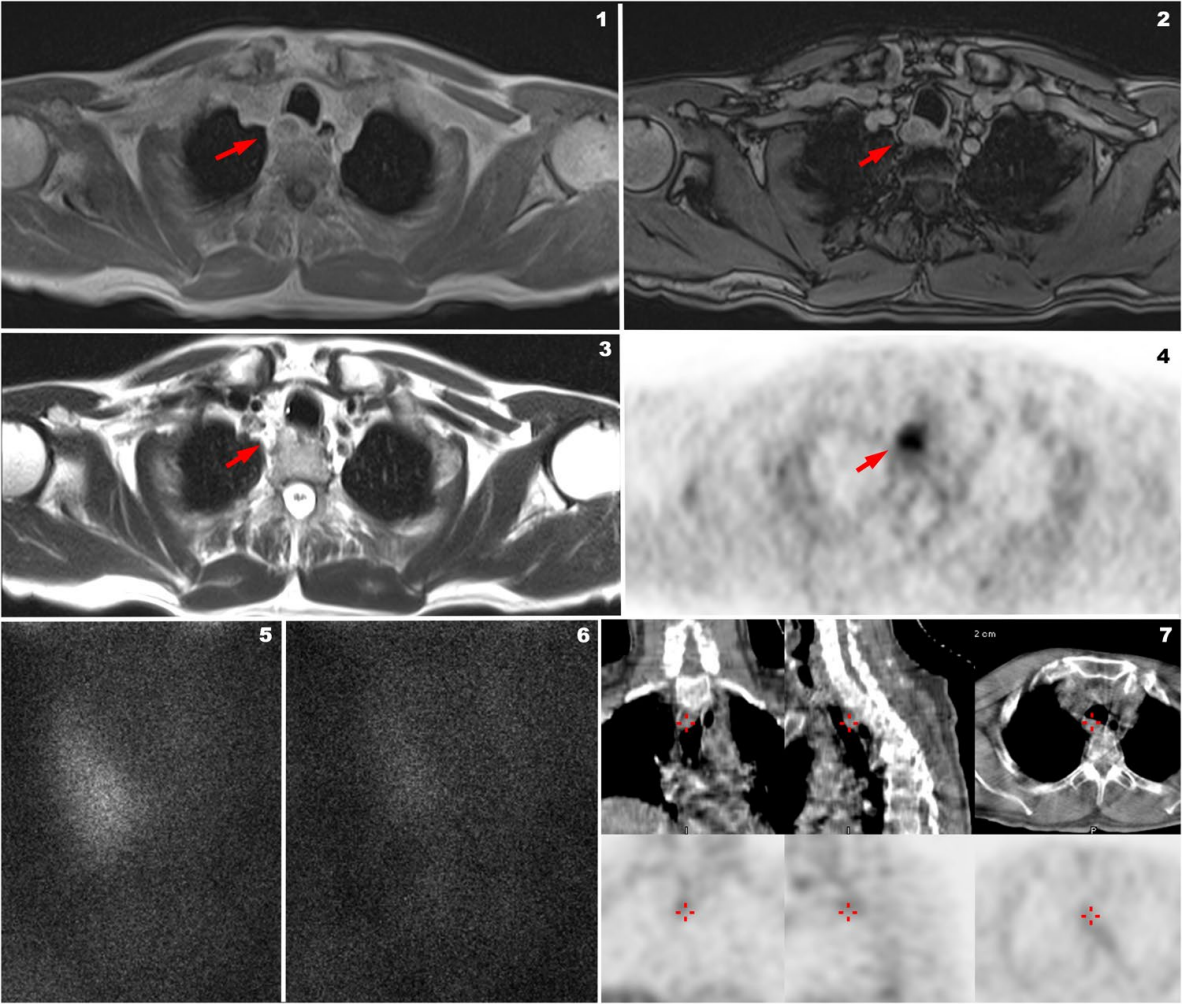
Example of positive 18F-FCH PET/MRI (Biograph mMR, Siemens Germany) and negative 99MTc-MIBI SPECT/CT (Infinia Hawkeye, GE Healthcare) performed at the University Hospital of Padova, Department of Medicine, Unit of Nuclear Medicine in a patient with hyperparathyroidism after left thyroidectomy (during a surgical procedure of left parathyroidectomy). MR axial Caipirinha in-phase (1) and MR axial Caipirinha out of-phase (2) demonstrating an ovoidal mass (red arrows) partially liquid at axial T2-Haste (3) with a very high posterior, paratracheal uptake of 18F-FCH PET/MR (4, red arrow). 99MTc-MIBI early after injection (5) and late after injection (6) phases of the same patient demonstrating no significant late retention of MIBI in the area revealed by 18F-FCH PET/MRI. SPET/CT of the same patient revealed only faint uptake (7: red cross) of MIBI in the paratracheal area revealed by 18F-FCH PET comparable to the background
